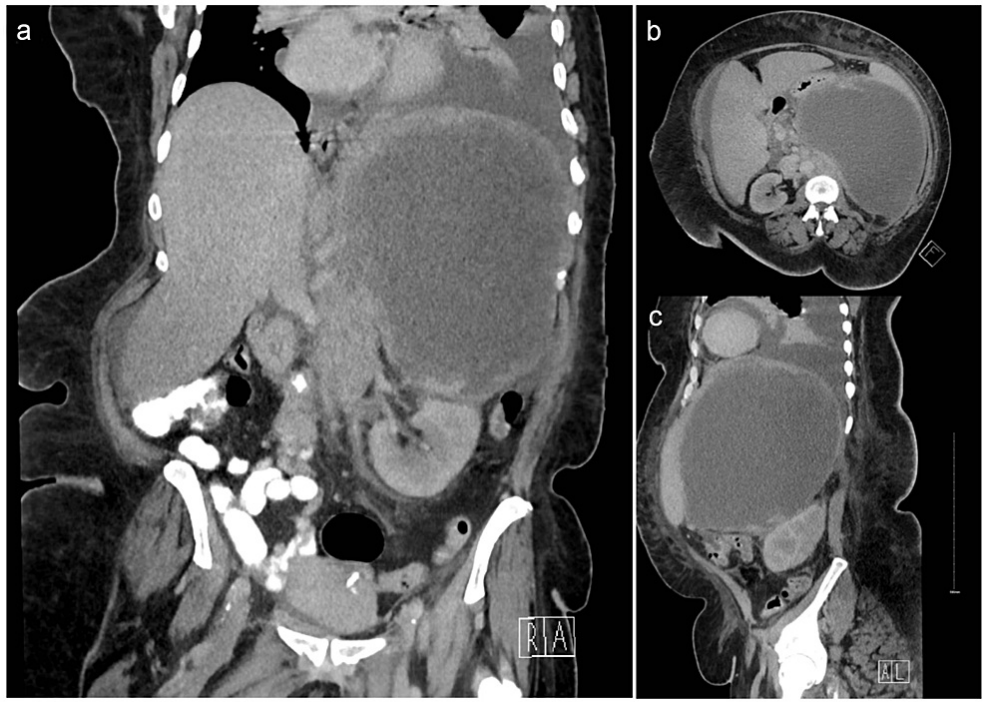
CT abdomen/pelvis with IV contrast with coronal (A), axial (B), and sagittal (C) views of the cystic retroperitoneal mass demonstrating displacement of retroperitoneal and intra-peritoneal structures. CT: computed tomography; IV: intravenous
